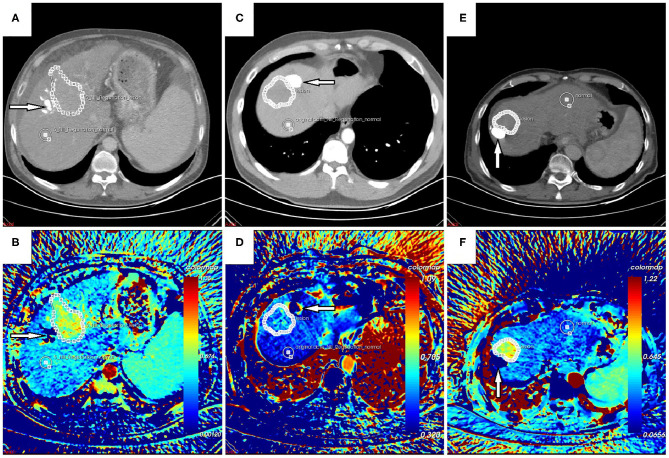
(A,B) Follow-up CT scan and arterial enhancement fraction (AEF) map of a 54-year-old male patient with no hepatitis background. (C,D) Follow-up CT scan and AEF map of a 48-year-old male patient with hepatitis B. (E,F) Follow-up CT scan and AEF map of a 66-year-old male patient with hepatitis B. They were all treated with transarterial chemoembolization (TACE) previously. The previous lesion with lipiodol deposition could be seen (arrow) on CT images, which should show as a “hollow zone” (B,F) on the AEF map despite some fake color (D) that was occasionally present due to tiny motion. Beside the “hollow zone,” a warmer region could be detected and was delineated as the “lesion” region of interest (ROI) even though it was difficult to recognize on CT images. Another ROI of “control” was delineated as well. The AEF mean value was significantly higher for “lesion” than for “control,” which indicated tumor recurrence, and they were confirmed by hepatic arteriography during the following TACE.
